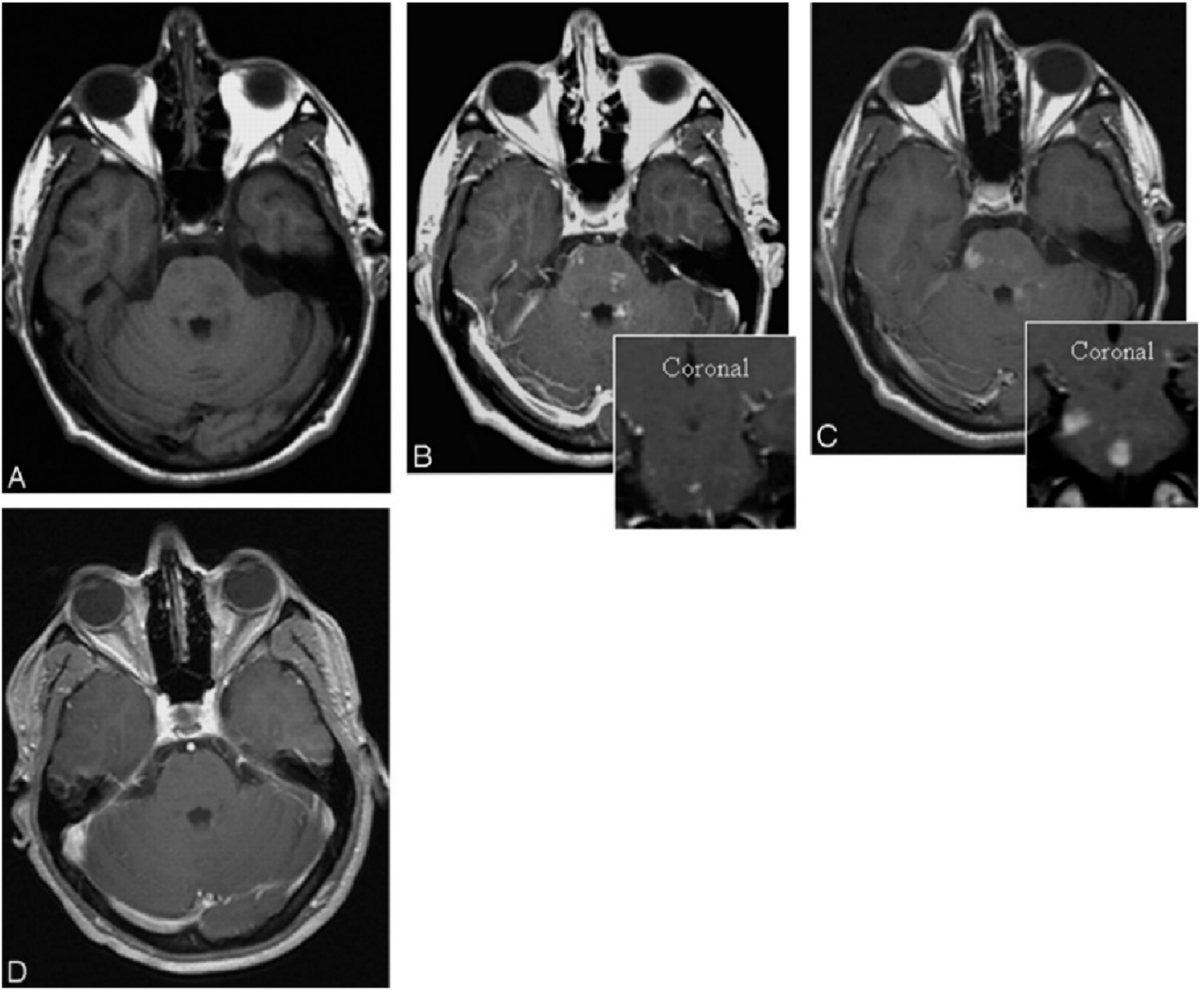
Patient with ADEM. Axial T1-weighted images without (A) and with (B) gadolinium (inset coronal) reveal faint and subtle enhancement in multiple brain stem lesions. Six days later (C), the same site exhibits significant, more pronounced, and larger enhancement with ferumoxtran-10 (inset, coronal). Three months later (D), the lesions no longer show enhancement on T1-weighted images with gadolinium.
